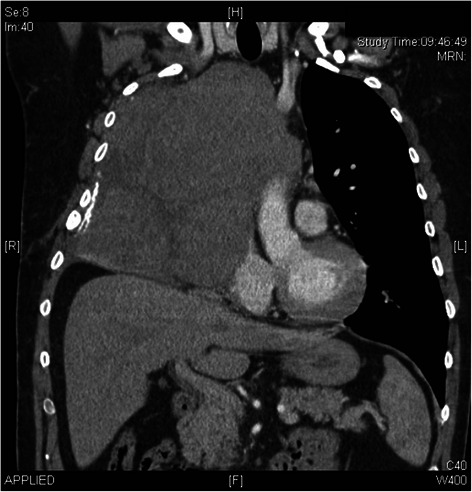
Preoperative computed tomography scan of patient 11. Carcinoid infiltrating right lung and Vena cava superior
